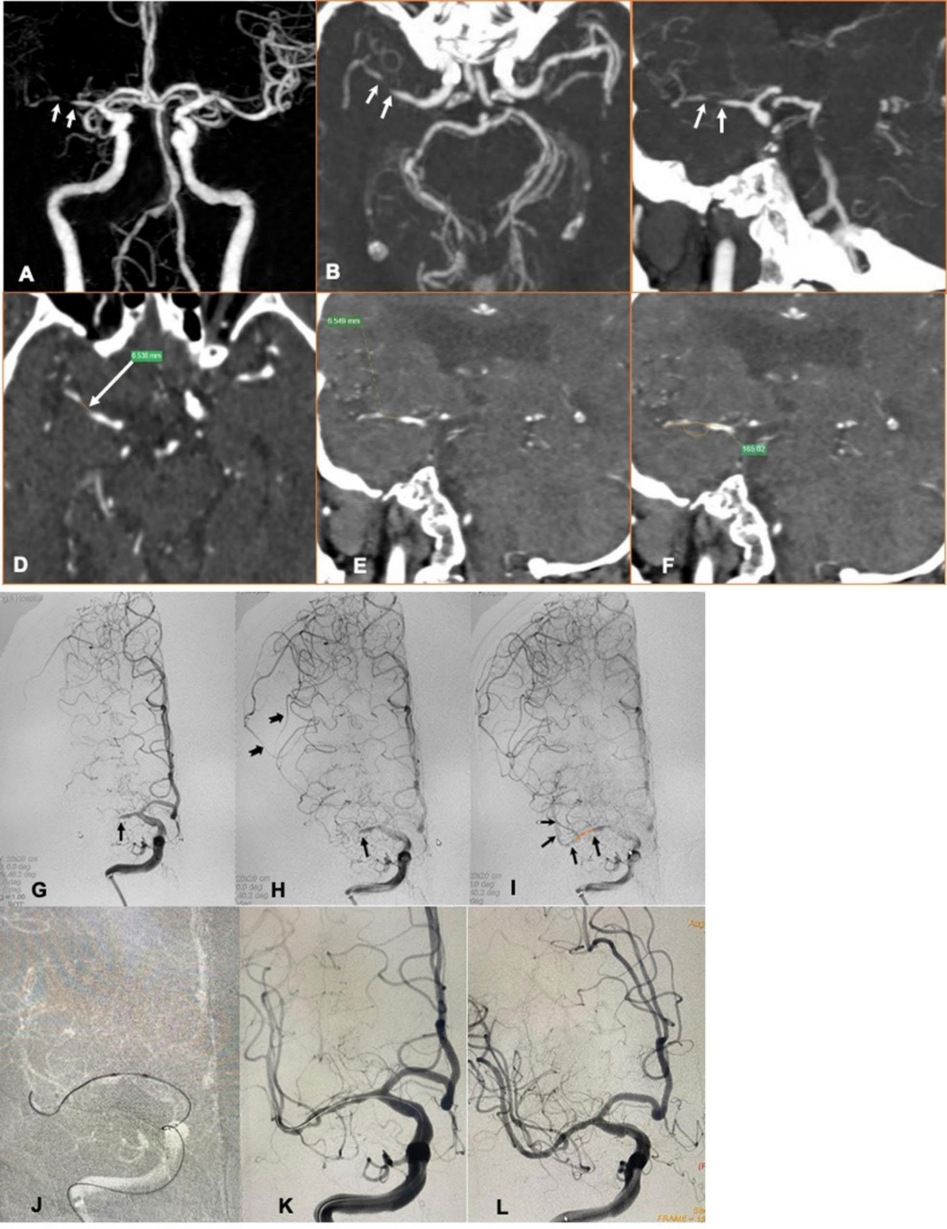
A patient with symptomatic middle cerebral artery (MCA) occlusion underwent endovascular recanalization that led to successful recanalization. A-C: Preprocedural computed tomography angiography (CTA) volume rendering (VR) reconstruction (A) and maximal intensity projection (MIP; B and C) images showed an interrupted continuity in the M1 segment of the right MCA (white arrow). D and E: Multiple planar reconstruction (MPR) of CTA images showed the total luminal filling defect at the occluded segment of the MCA; the occlusion length was 6.5 mm. F: MPR of CTA images showed the occluded segment made a 15° turn from the proximal to the distal M1 segment. G-I: Digital subtraction angiography (DSA) confirmed occlusion of M1 segment of the right MCA (G), a tapered stump of the occluded segment (black arrow), and a slow antegrade flow through the occluded segment (TIMI grade 1) and visualization of the vascular bed at the distal end of the occlusion (black dovetail arrow), as well as retrograde blood flow (black dovetail arrowhead) from the anterior cerebral artery on the midarterial-phase (H) and late arterial-phase images (I). J: After successful navigation of the microwire and microcatheter through the occlusion, a 1.5 × 10 mm rapid-exchange balloon (Neuro LPS™, Sinomed, Tianjin, China) was positioned to the occluded segment (K), after which the lesion was predilated with 1.5 × 10 mm and 2.25 × 10 mm balloons and a 2.5 × 18 mm LEO baby stent was implanted. L: Postprocedural DSA demonstrated technically successful recanalization with TICI grade 3
